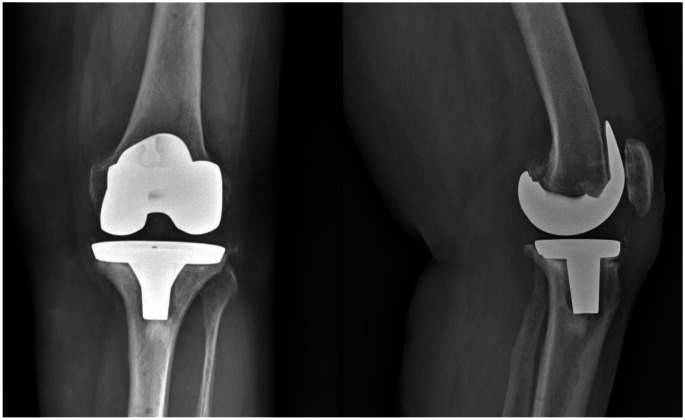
X-rays of a 72-year-old individual with Parkinson’s disease, taken one year following total knee arthroplasty (TKA) performed using a functional alignment technique. At the follow-up, the images revealed implant loosening, which was associated with poor functional and clinical outcomes and ultimately required a two-stage implant revision
